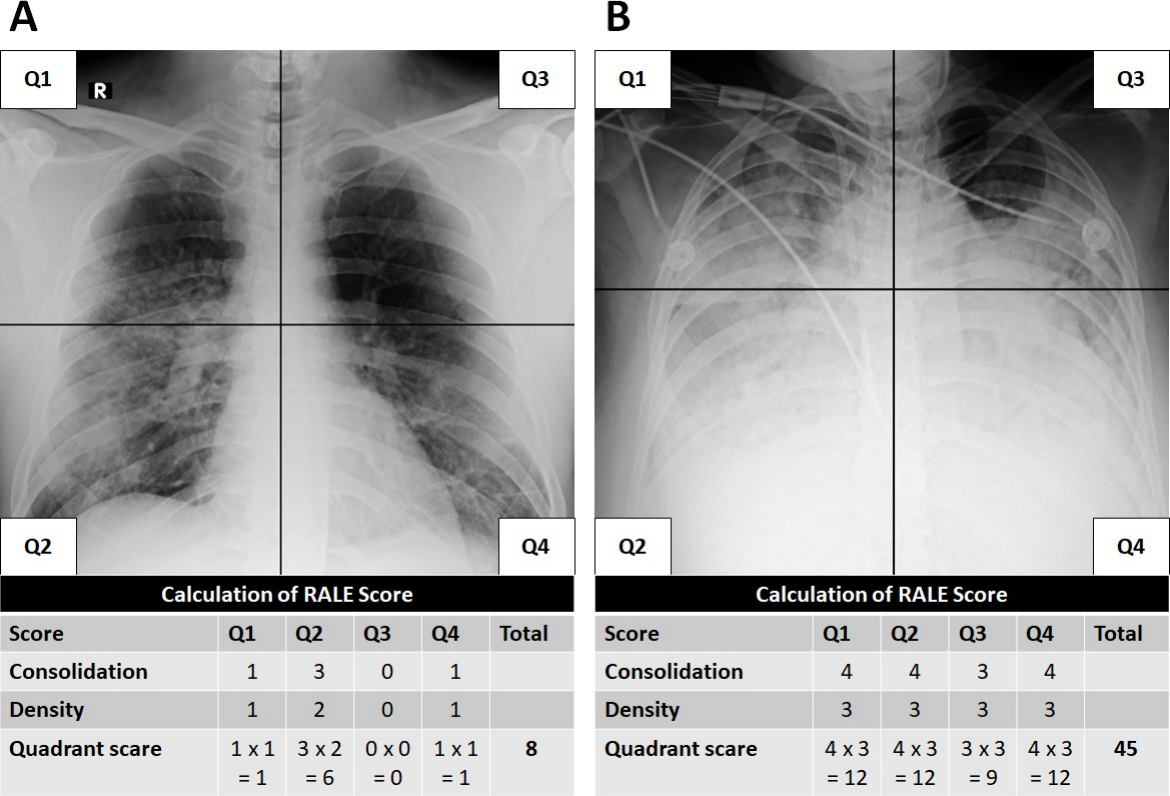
Examples of RALE scoring in COVID-19 positive patients. (A) Initial frontal chest radiograph in a 32-year-old male from the survivor cohort with total RALE score of 8. (B) Initial frontal chest radiograph in a 48-year-old female from the deceased cohort with total RALE score of 45.
